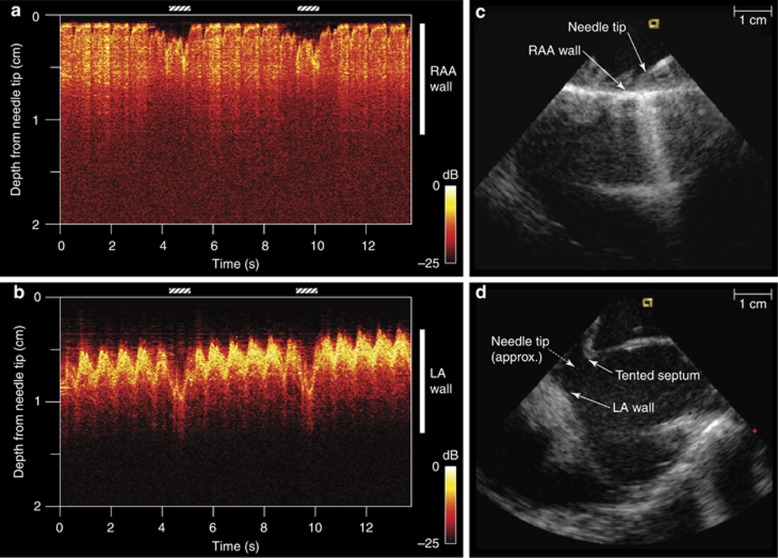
All-optical ultrasound imaging (M-mode) before (a) and after (b) the perforation of the cardiac septum. Corresponding needle tip positions were identified with a commercial intracardiac echocardiography catheter (c and d). With the needle tip positioned at the right atrial appendage wall, imaging depths extended more than 1 cm into tissue a. Cardiac motion, which manifested as slight deviations of the tissue surface relative to the needle tip, was more prominent during mechanical ventilation (diagonal bars). Immediately following perforation and entrance into the left atrium (LA), pronounced LA wall cardiac motion was readily apparent b.
